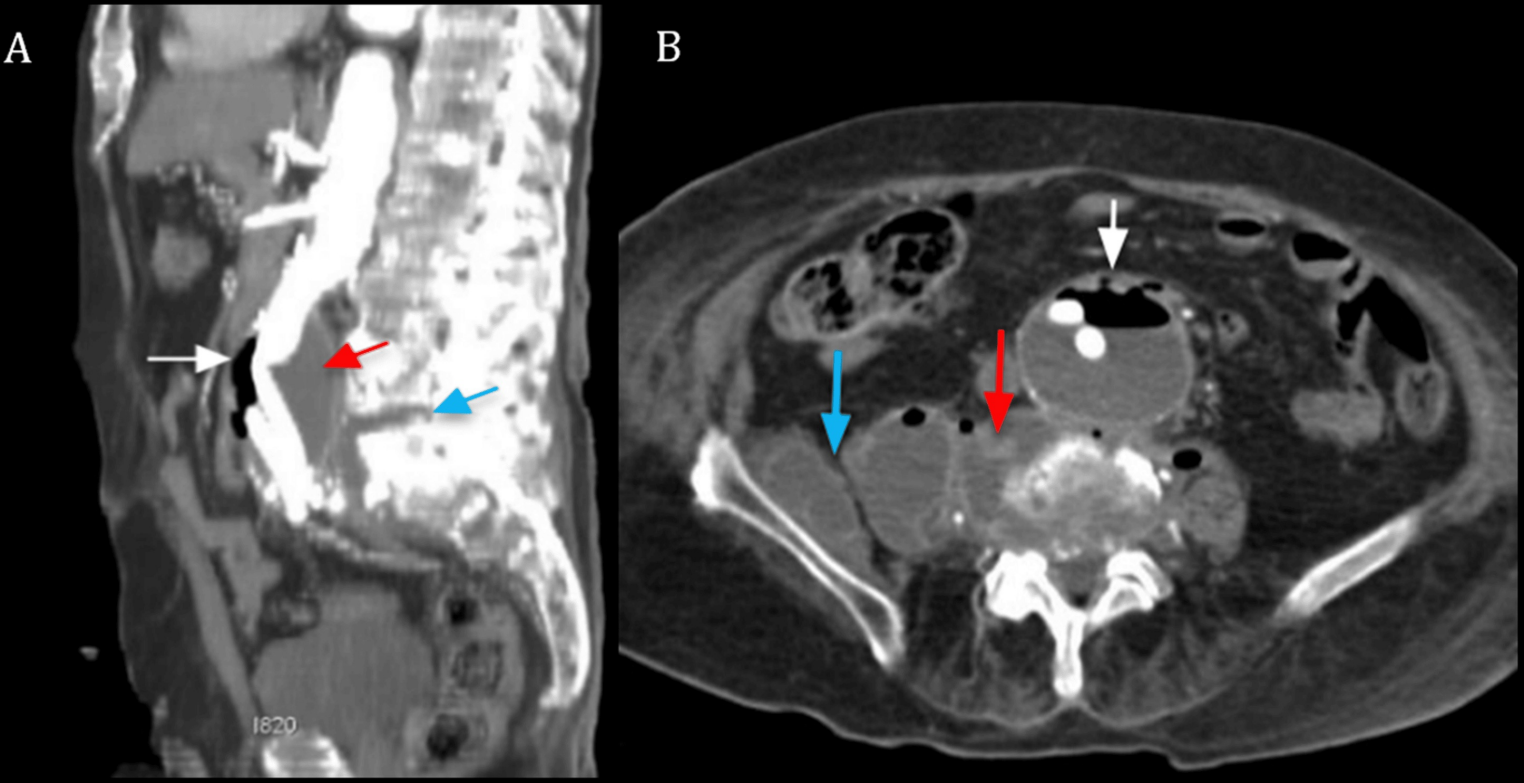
Abdominal CT angiography Image A. MIP sagittal CT view depicts an abdominal aortic aneurysm located below the renal arteries, with an air-fluid level (white arrow). Posteriorly, a paravertebral collection (red arrow) is identified, associated with L5-S1 disc destruction, and a collection (blue arrow), indicative of spondylodiscitis, in close proximity to the aneurysm. Image B. Axial CT slice demonstrates an anterior communication between the aneurysm’s anterior wall and an adjacent small intestinal loop (white arrow). Posteriorly, the paravertebral collection (red arrow) extends into the surrounding soft tissues, involving the right psoas and iliacus muscles (blue arrow).
